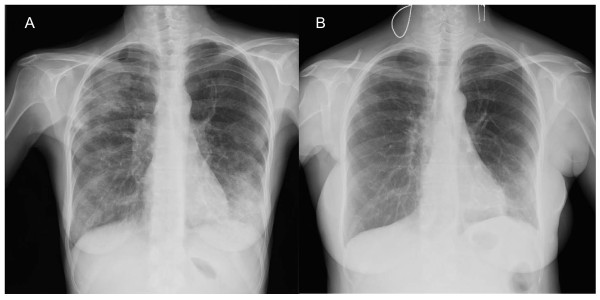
Chest radiograph of case 1. A: At presentation, diffuse bilateral pulmonary infiltrates predominant to upper right and lower left lobes. B: Major improvement after 2 years of treatment.
